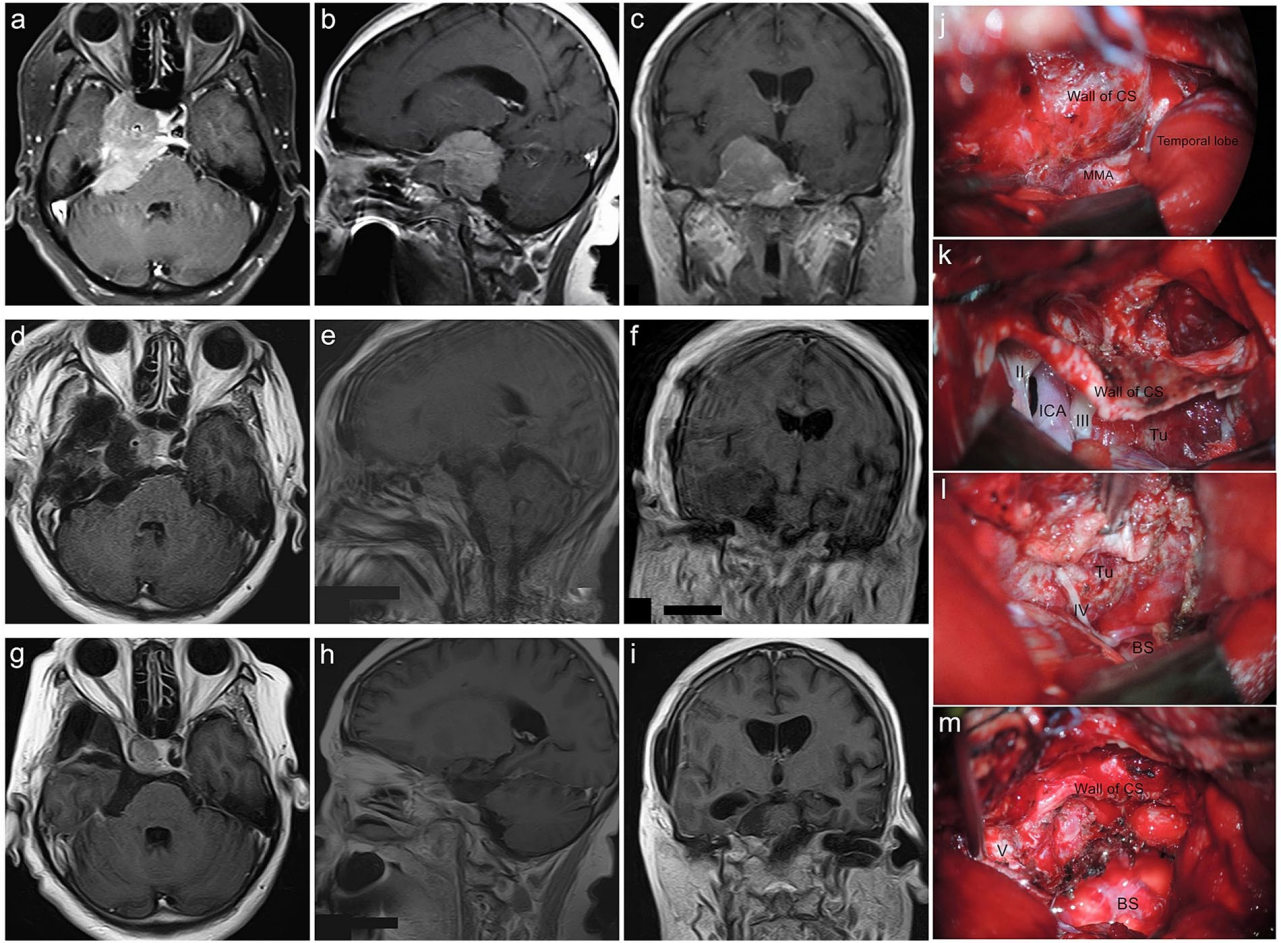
Sphenopetroclival subtype I case. A 40-year-old female presented with facial numbness, altered ocular motility and hearing impairment for 48 months. The preoperative KPS score was 90. She was achieved GTR with the PTCA of palsy in CN III and VI and intracranial infection. The postoperative KPS score was 50. With a follow-up of 45 months, she participated in normal activities and had a KPS score of 90 without recurrence. (a–c) Preoperative MRI T1 contrast axial, sagittal, and coronal images. (d–f) Postoperative MRI T1 contrast axial, sagittal, and coronal images. (g–i) Follow-up MRI T1 contrast axial, sagittal, and coronal images. (j–m) Intraoperative figures demonstrating steps of sphenopetroclival subtype I resection via the PTCA. (j) Exposure of the wall of the cavernous sinus within part of tumor and middle meningeal artery after temporal lobe retraction. (k) Coagulation of the middle meningeal artery, incision of wall of cavernous sinus to remove the tumor within the sinus, then incision of the dura mater to expose the subdural structures and tumor. (l) Subdural tumor resection between the multiple intervals of the neurovascular structures. (m) Total tumor resection and keeping well the brain stem and neurovascular structures integrity. MMA, Middle Meningeal Artery; ICA, Internal Carotid Artery; Tu, Tumor; BS, Brainstem.
